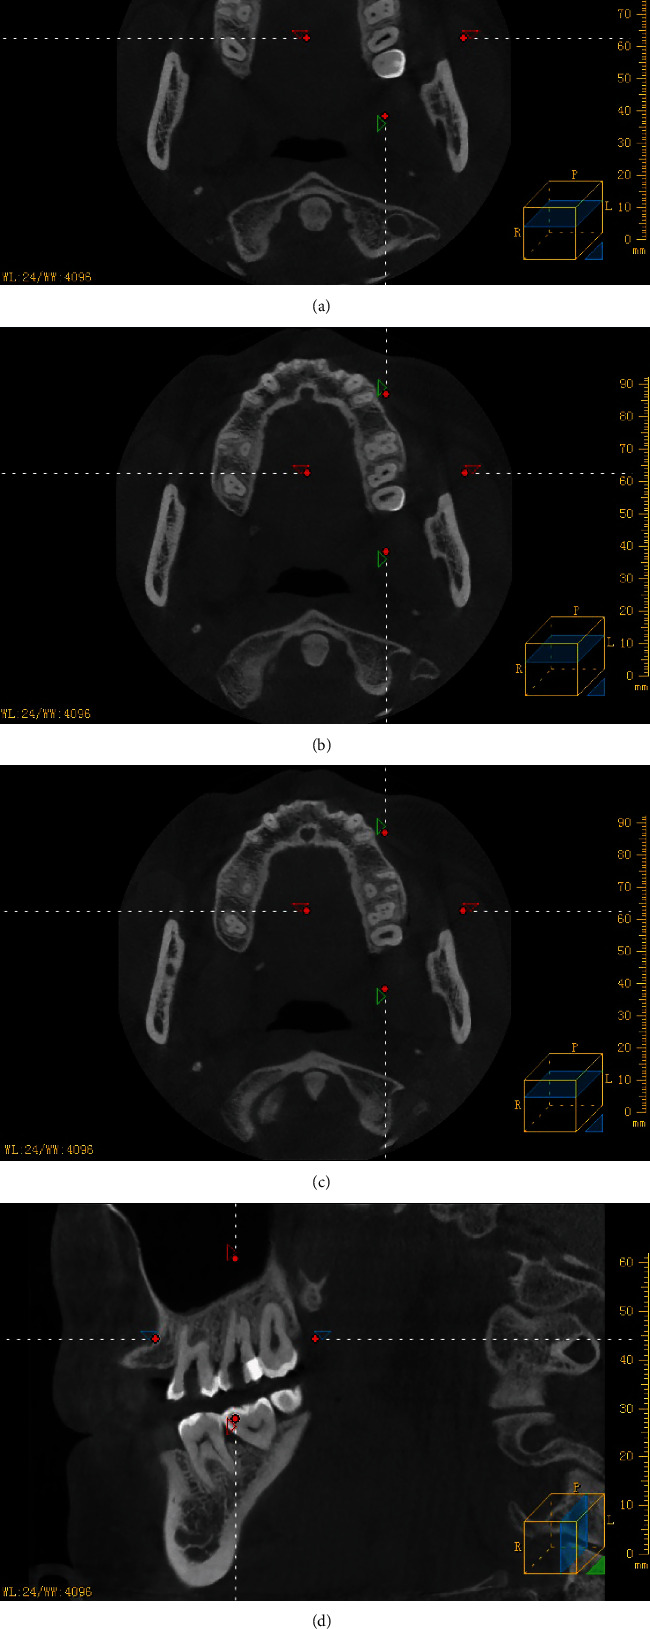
Cone-beam computed tomography images. Horizontal sections at (a) orifice level, (b) midroot level, (c) apical level, and (d) sagittal view reveal a type 1–3 canal.
